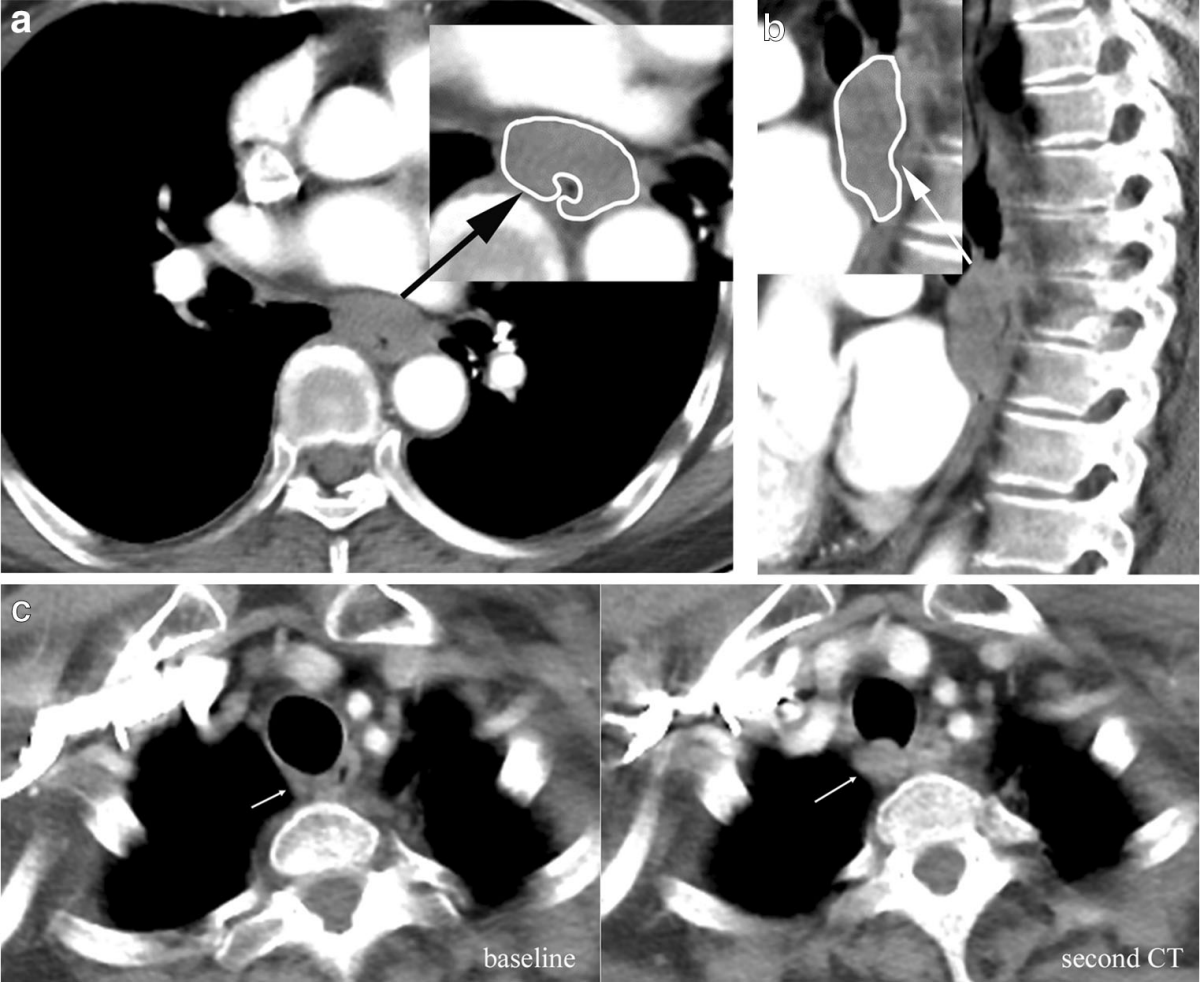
Regions of interest (ROIs). The ROIs (white circles) were manually drawn to encompass the tumour on axial image (a) on baseline CT and the second CT, and on sagittal image (b) avoiding necrosis, vessels, haemorrhage, and oesophageal lumen. c An enlarged small lymph node (ESLN) on the second CT. Baseline CT revealed a small lymph node with a short diameter of 4.4 mm in the right para-oesophageal region (white arrow), and CT after two cycles of induction chemotherapy showed an enlarged lymph node with a short diameter of 9.5 mm in the right para-oesophageal region (white arrow)
